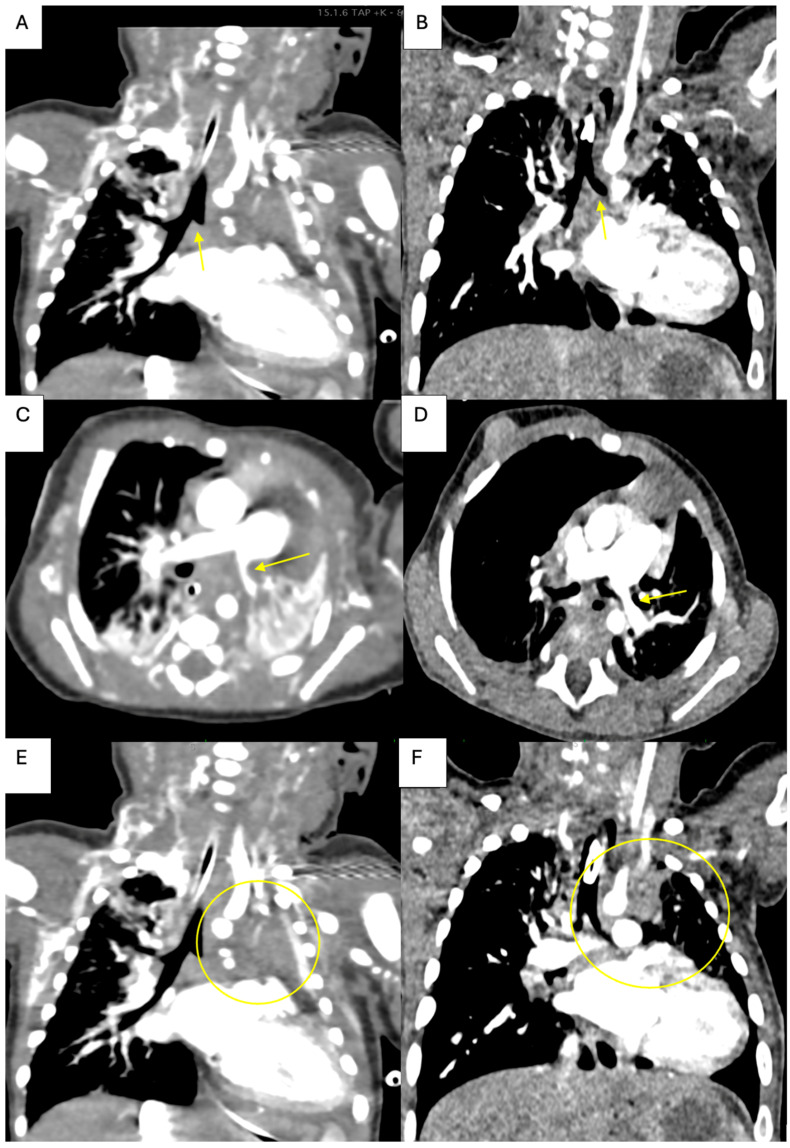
Chest CT comparison at 12 months vs. preoperative; (A, arrow) preoperative severe proximal left bronchus stenosis; (B, arrow) moderate left main bronchus proximal stenosis at 12 months; (C, arrow) preoperative severe proximal left pulmonary artery stenosis; (D, arrow) moderate proximal left pulmonary artery stenosis at 12 months; (E, circle) preoperative superior mediastinal tumor mass; (F, circle) no tumor mass can be seen at the level of superior mediastinum at 12 months.
